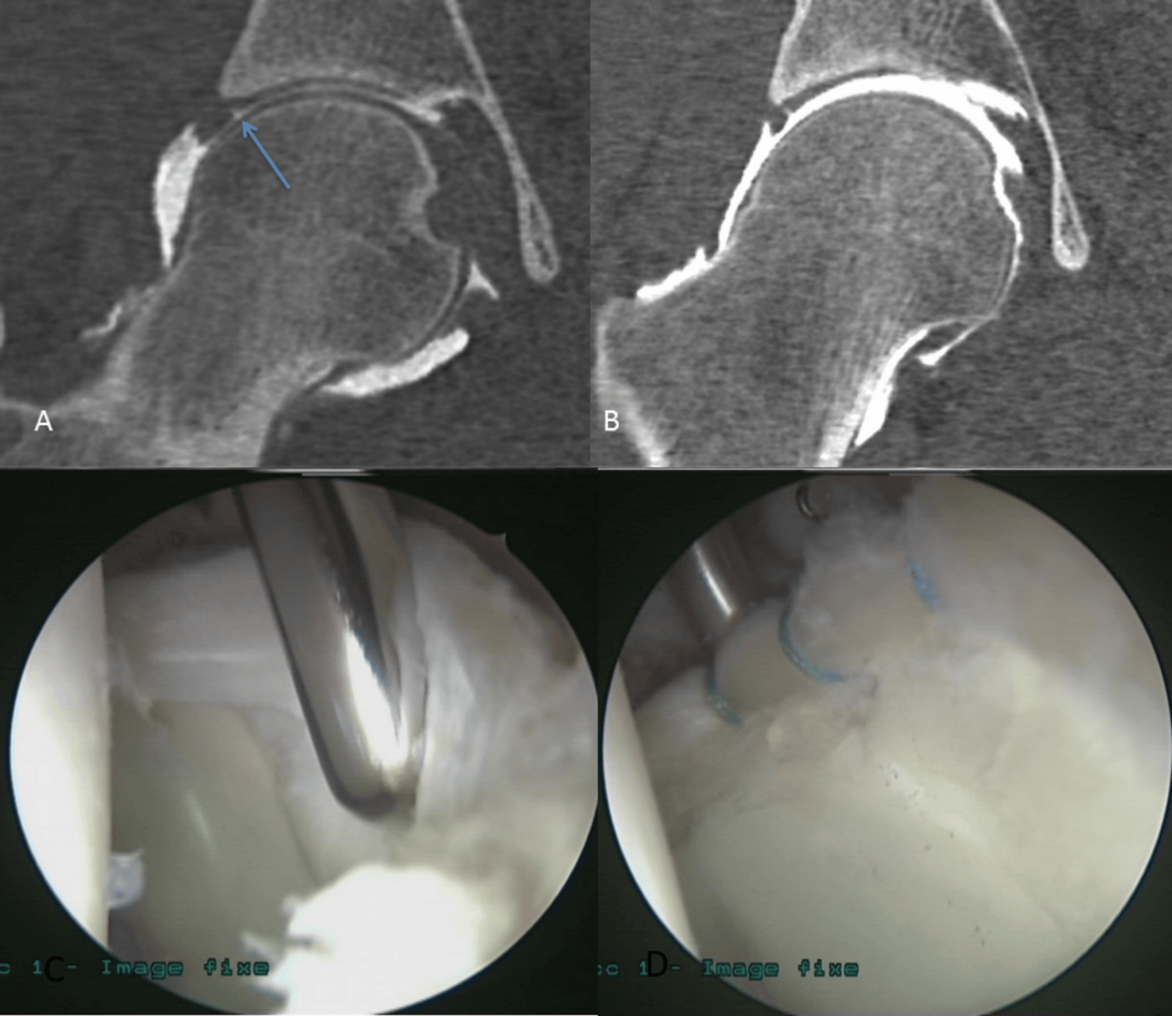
Labral lesions CTA of the right hip with labral-chondral separation without (A) and with (B) traction in the same patient 1 (MAHORN grade 1). These coronal reformations of CTA of the right hip show better labral-chondral separation assessment (arrow) with traction than without traction in the same patient. Arthroscopy comparison before (C) and after (D) suture treatment in patient 2. CTA: computed tomography arthrography, MAHORN: Multicenter Arthroscopy of the Hip Outcomes Research Network
